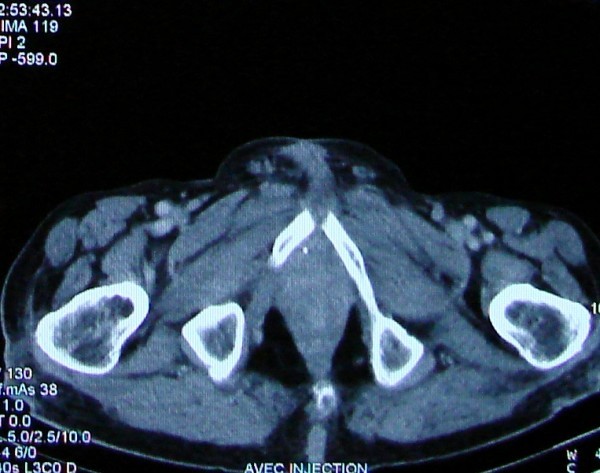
Pelvic computed tomography scan performed after the end of chemotherapy shows complete radiological response of the ano-rectal tumour.
